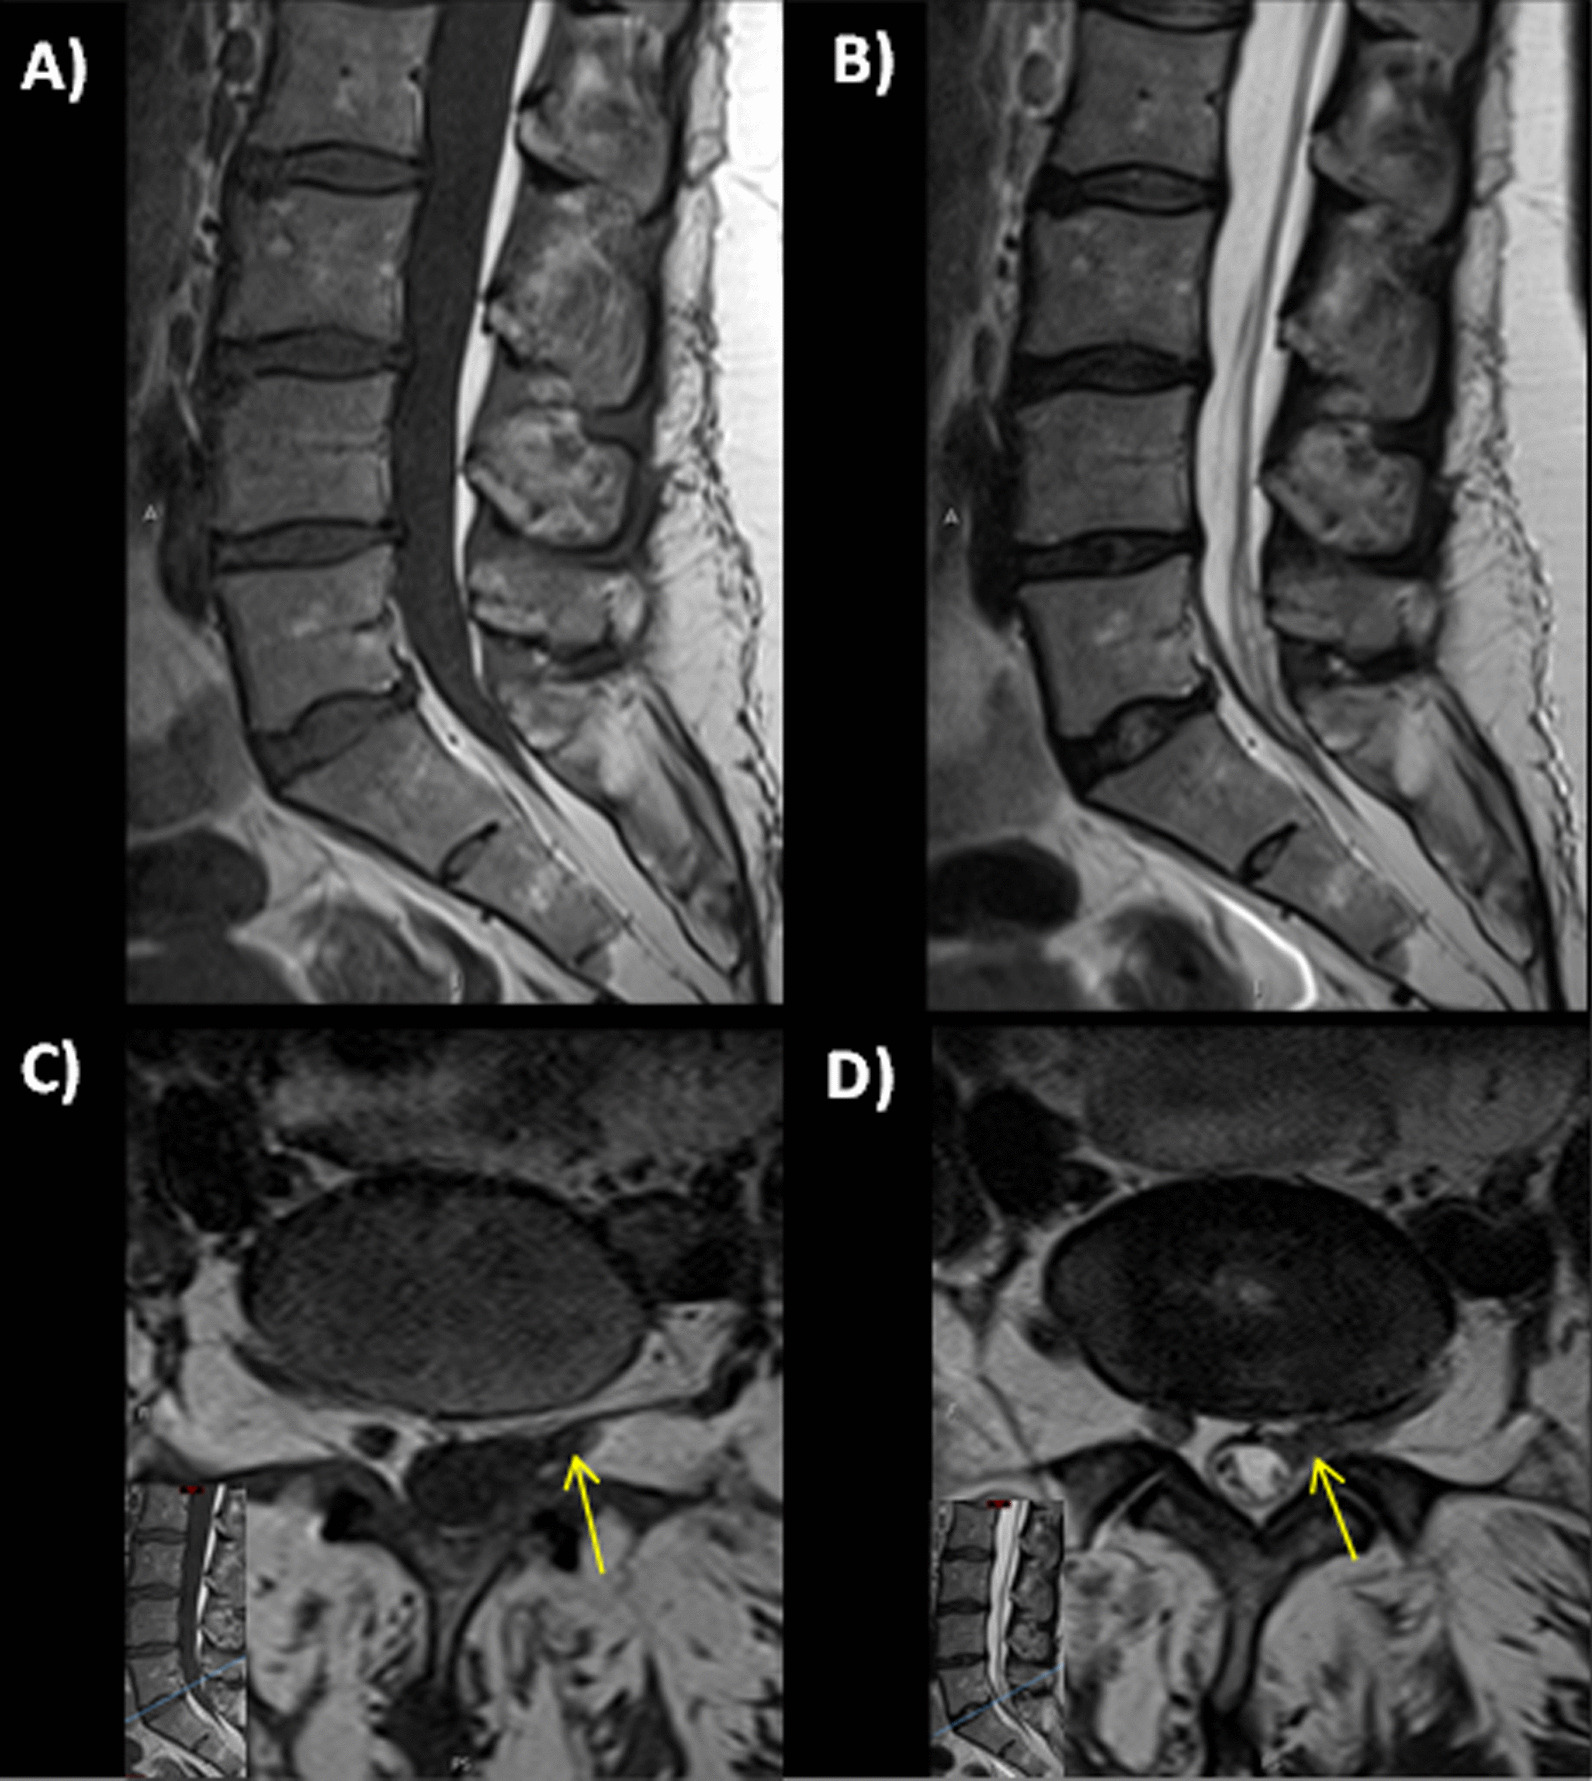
Magnetic resonance imaging lumbar spine images 4 months later; A Sagittal T1-weighted; B Sagittal T2-weighted; C Axial T1-weighted; D Axial T2-weighted. Follow-up study performed 4 months later shows clearance of the intradural blood clot with minor residual clumping of the cauda equina nerve roots within the thecal sac (arrow)
